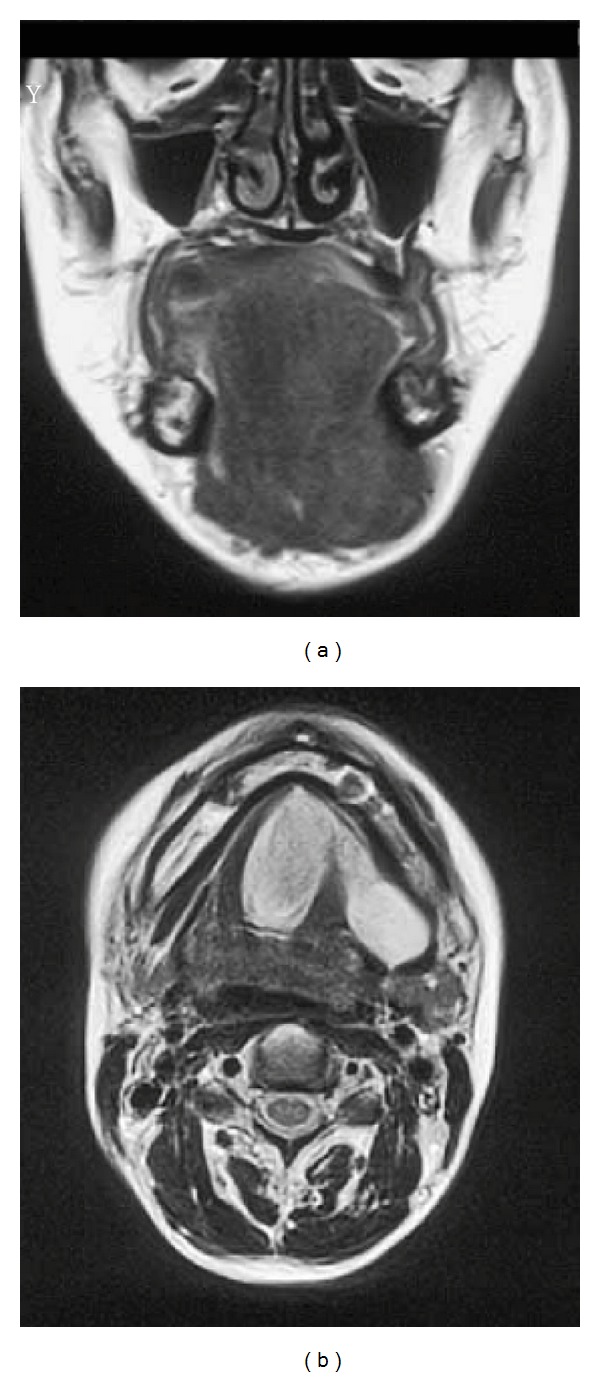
MRI images. Coronal T1-weighted image (a) and axial T2-weighted image (b). MRI revealed that the mass showed a homogenous low signal intensity on the T1-weighted image and high signal intensity on the T2-weighted image.
